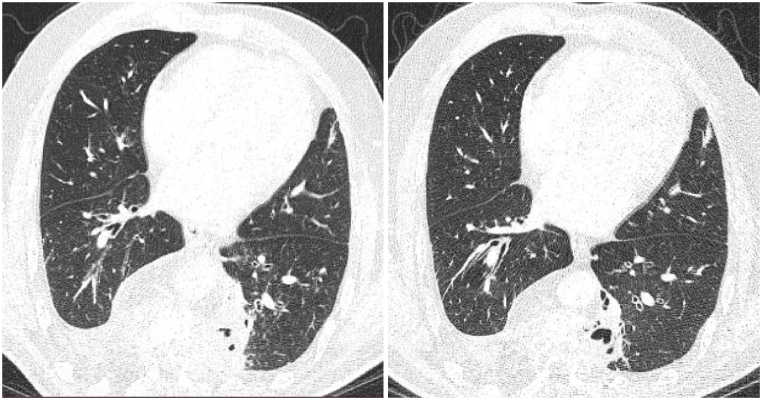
CT scan cross section at T9 vertebra with a parenchymal analysis (2020 on the left and 2022 on the right).
